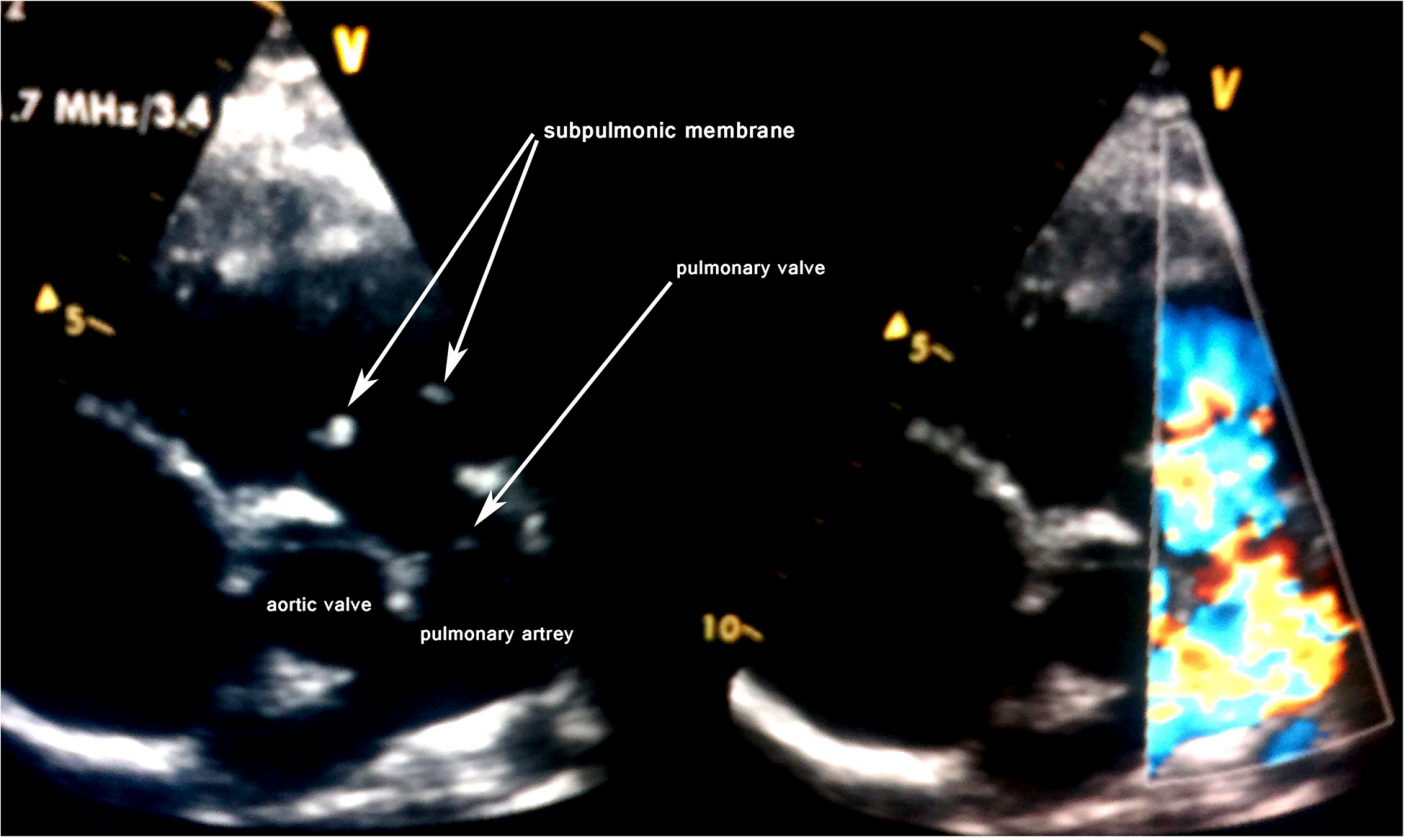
Two-dimensional and color flow echocardiographic frames showing discrete membrane below the pulmonary valve (post balloon dilation of the pulmonary valve).
